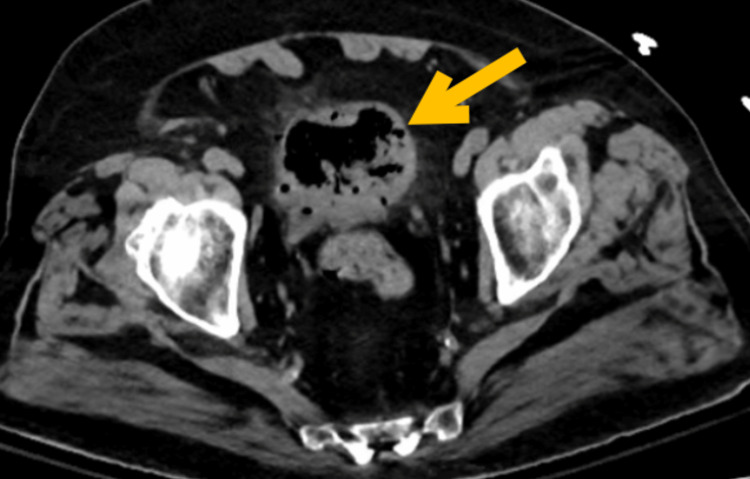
Non-contrast CT of the abdomen and pelvis (axial section through the urinary bladder). Diffuse emphysema throughout the bladder wall with surrounding stranding (arrow). CT: computed tomography
